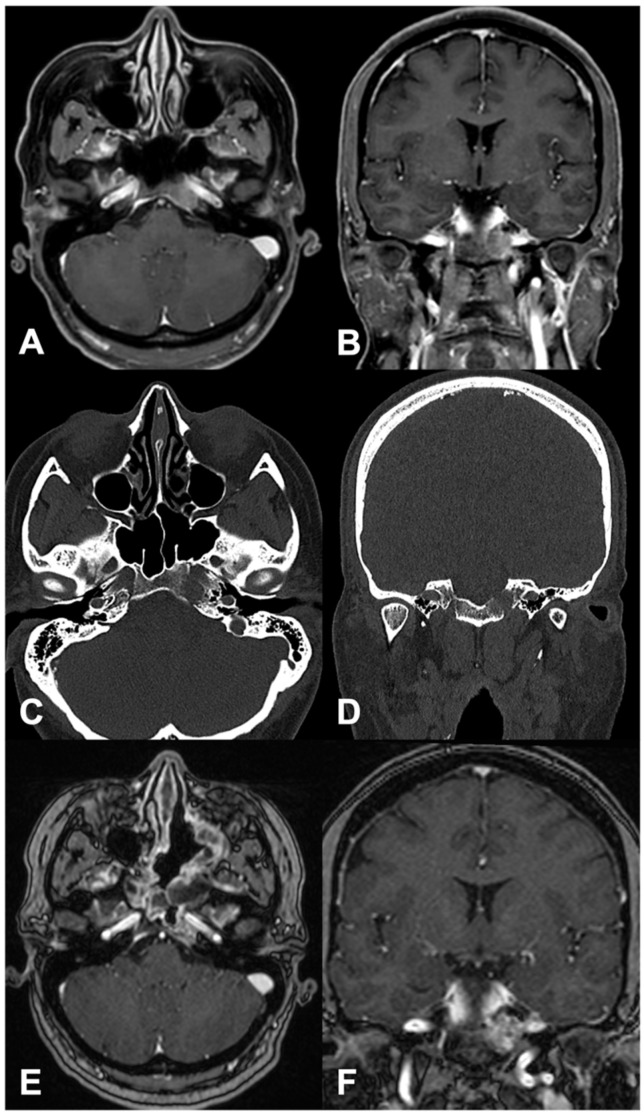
Preoperative images of case #4 (axial and coronal T1-weighted contrast-enhanced MRI, (A,B); axial and coronal bone window thin-slice CT, (C,D)). The neuroimaging shows a contrast-enhancing lesion on the left side of the clivus, lying posteriorly to the left intrapetrous ICA (A). The CT reveals the lesion to be osteolytic, with cortical bone interruption anteriorly and posteriorly, suggesting its invasiveness of the posterior cranial fossa and carotid canal (C). The 3-month postoperative images (axial and coronal T1-weighted contrast-enhanced MRI, (E,F)) show the partial resection of the medial part of the neoplasm.
